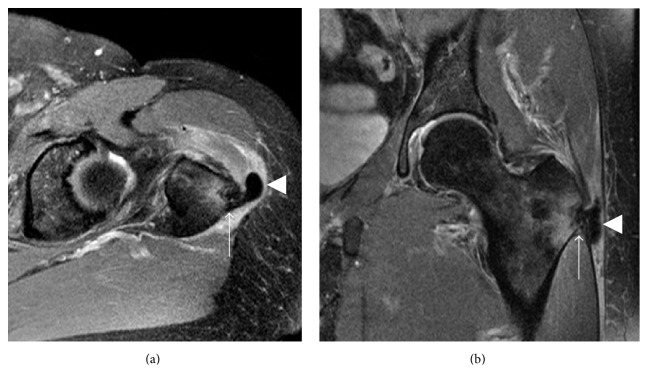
Calcific bursitis with osseous erosion. (a) and (b) Axial and coronal MRI images show a large conglomeration of soft tissue calcification within the greater trochanter bursa with surrounding soft tissue edema (arrowheads). The calcifications about the greater trochanter and erode the cortex with reactive edema of the adjacent bone marrow (arrows).
